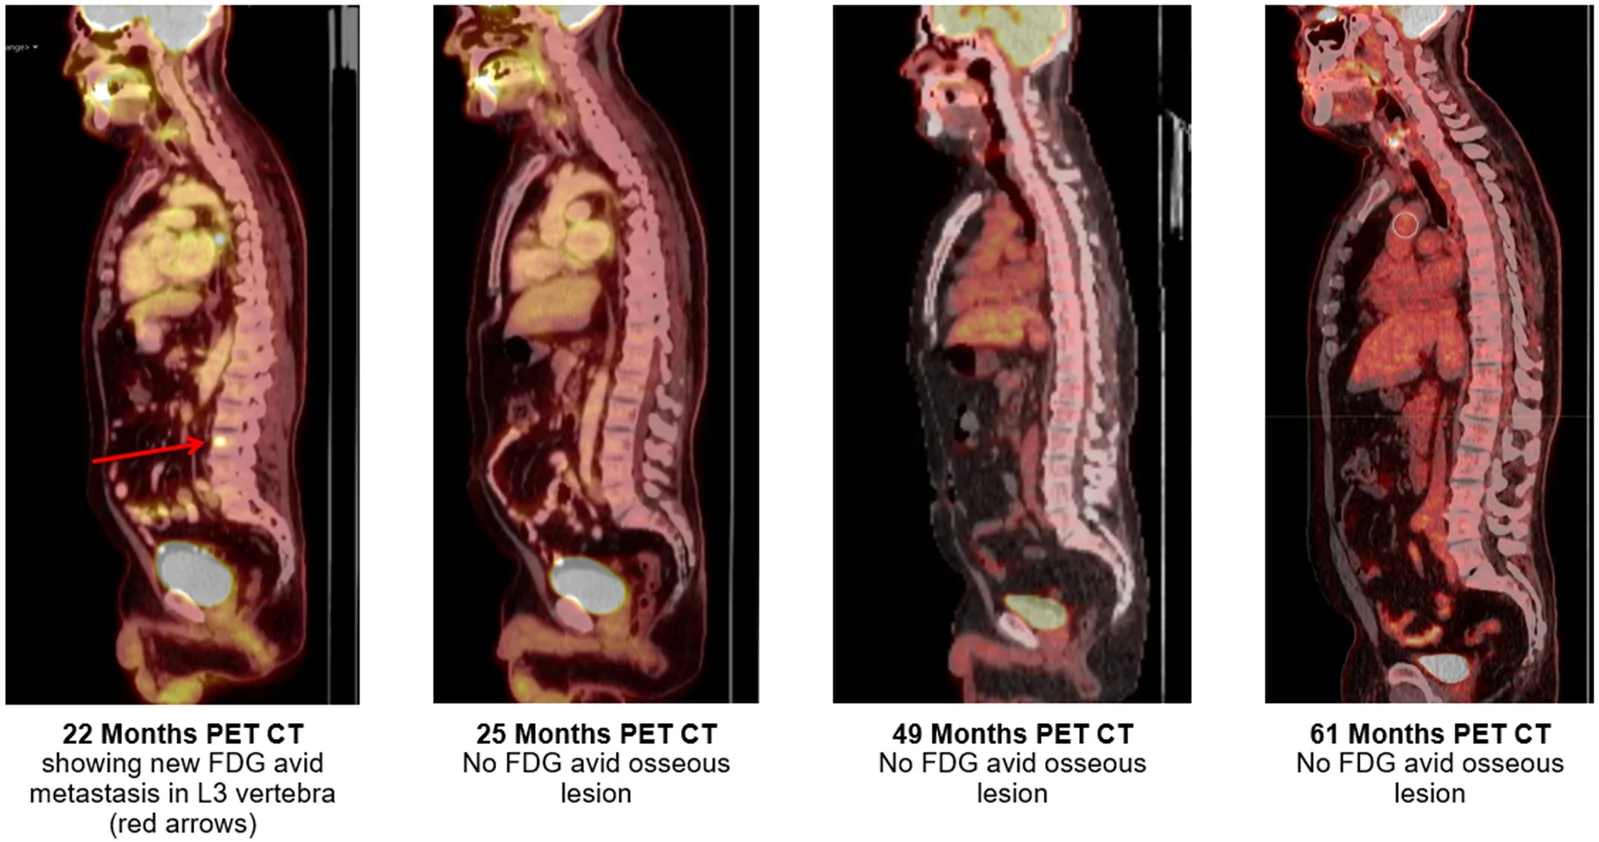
Sagittal view of PET CT demonstrating the FDG avid metastasis in L3 vertebra followed by metabolic resolution and sclerosis of the L3 lesion.
